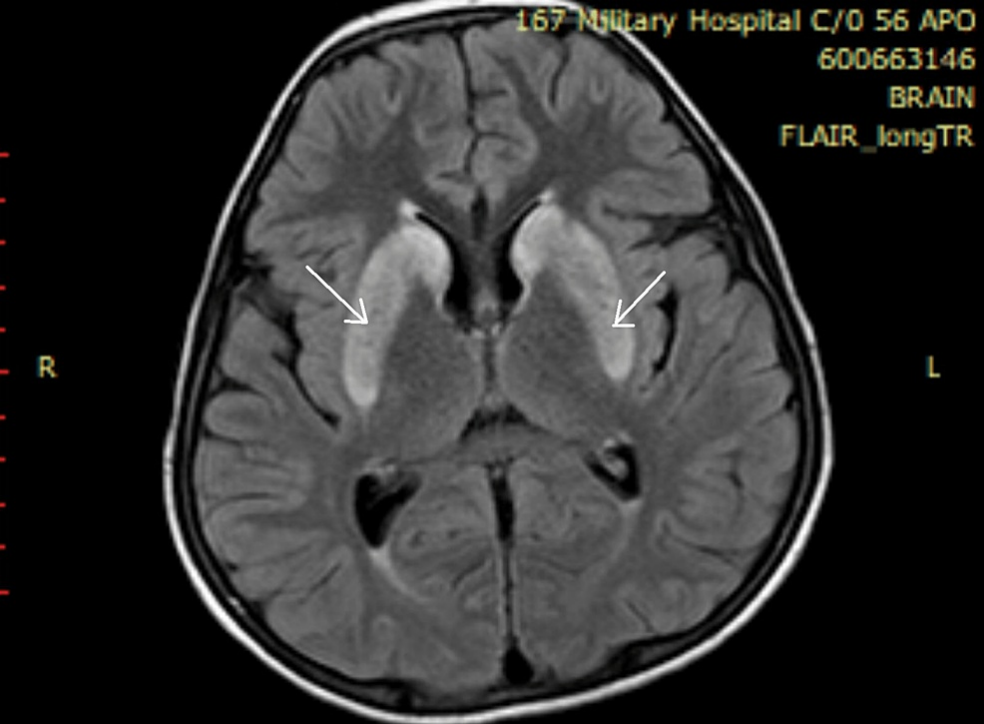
Hyperintensity in basal ganglia as seen on MRI FLAIR MRI: Magnetic Resonance Imaging
